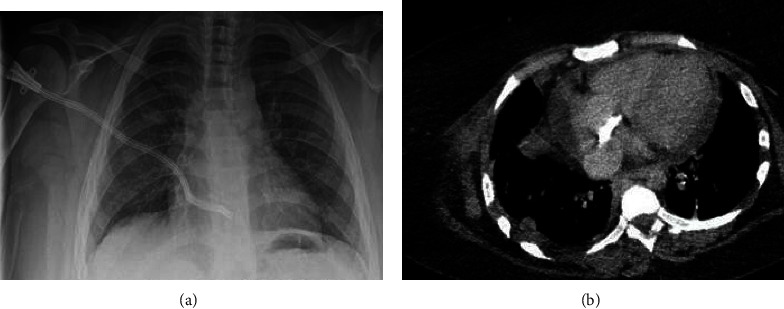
(a) Chest radiography showing the ATDC. (b) CT-scan showing the catheter location at the right atrium.
